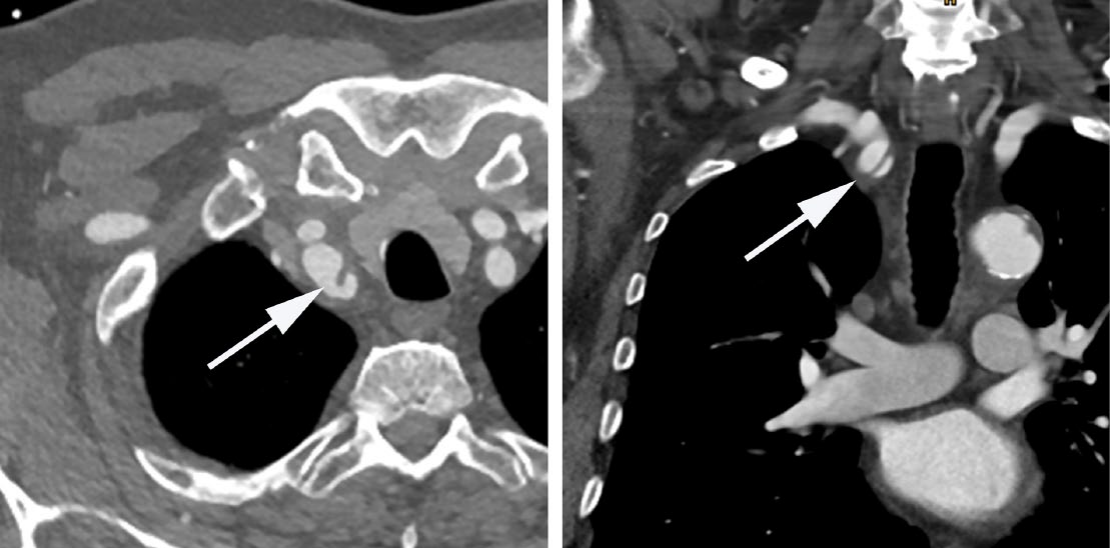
Computed tomography showing dissection of the brachiocephalic trunk (arrows) with sagittal and coronal views.
